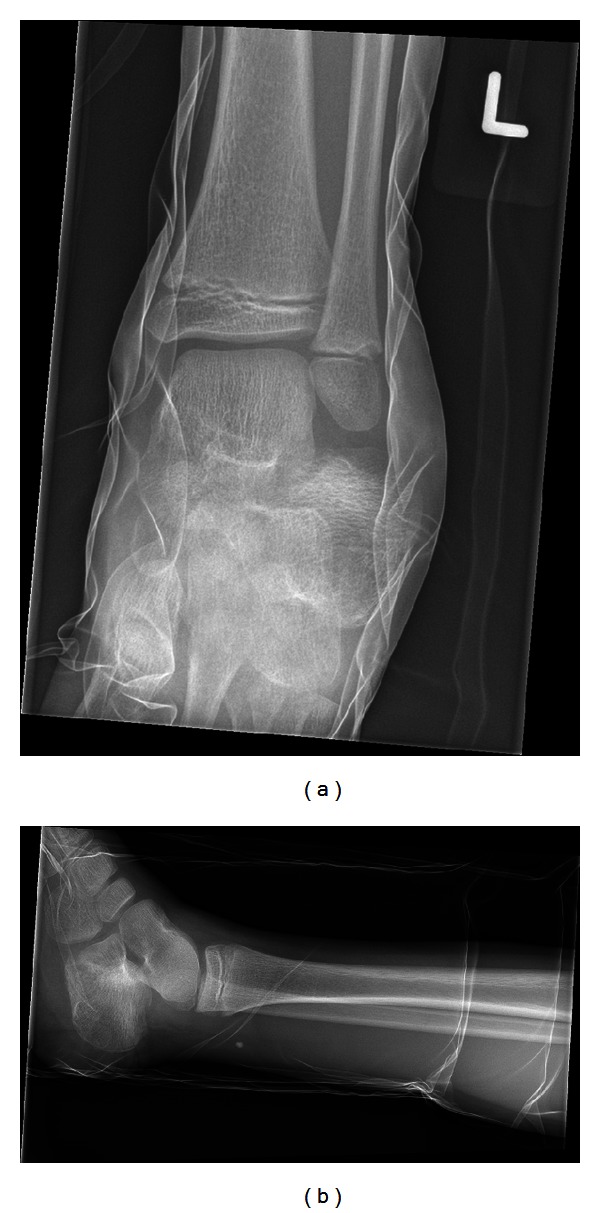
(a) Anteroposterior radiograph displaying two-part extra-articular calcaneal fracture. (b) Lateral radiograph displaying two-part extra-articular calcaneal fracture.
